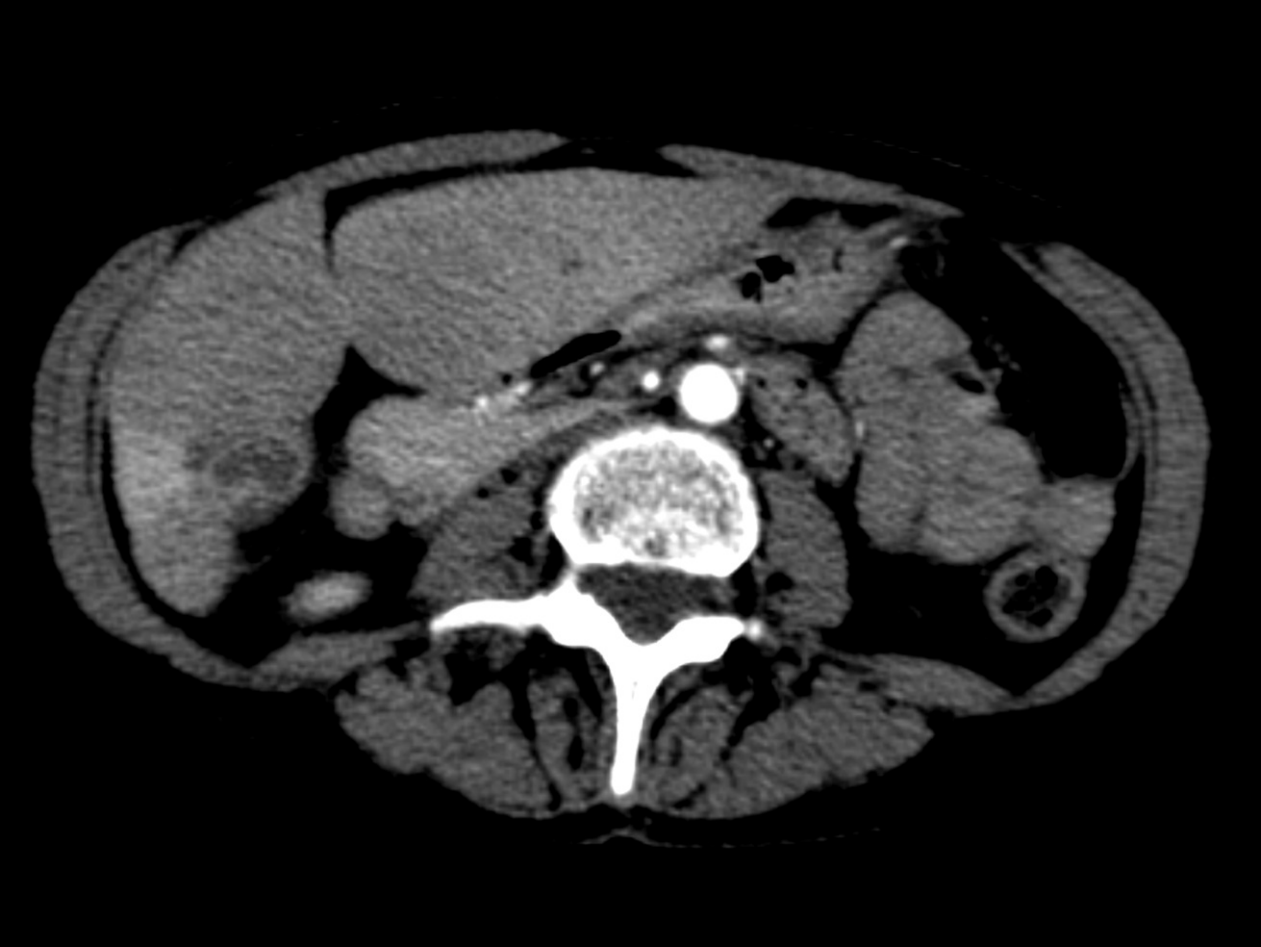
Representative axial slice from CT obtained three months after second SIRT procedure
